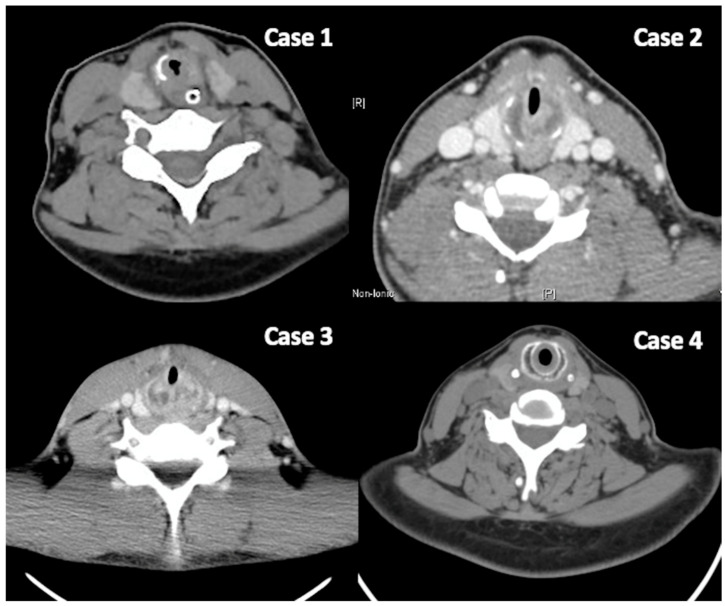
CT images revealed a thickened and edematous subglottic mucosa, along with destruction and deformation of the cartilage, indicating disease-induced damage.
